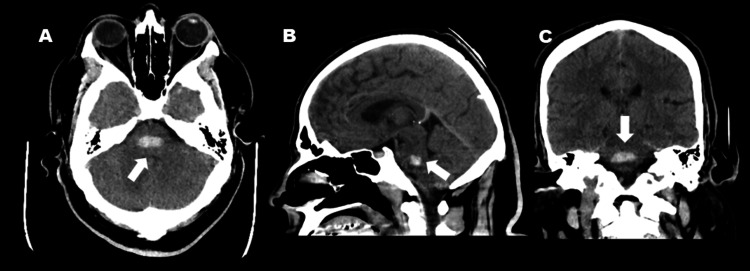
Acute hemorrhage in the pons. The axial (A) and sagittal (B) and coronal (C) of the initial brain computed tomography showed an acute phase hemorrhage (2.1 cm x 9 mm x 1.1 cm) of the pontine tegmentum (arrows).
